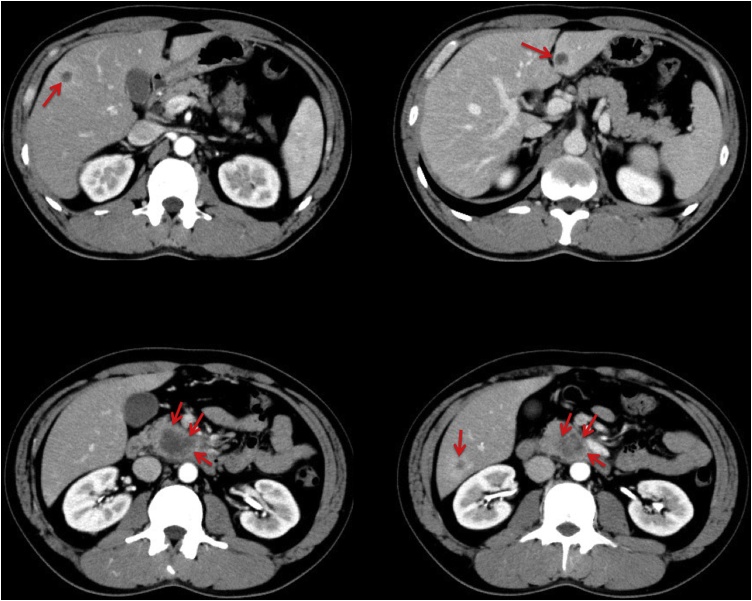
Enhanced computed tomography scan demonstrates pancreatic head cancer and multiple liver metastases.
